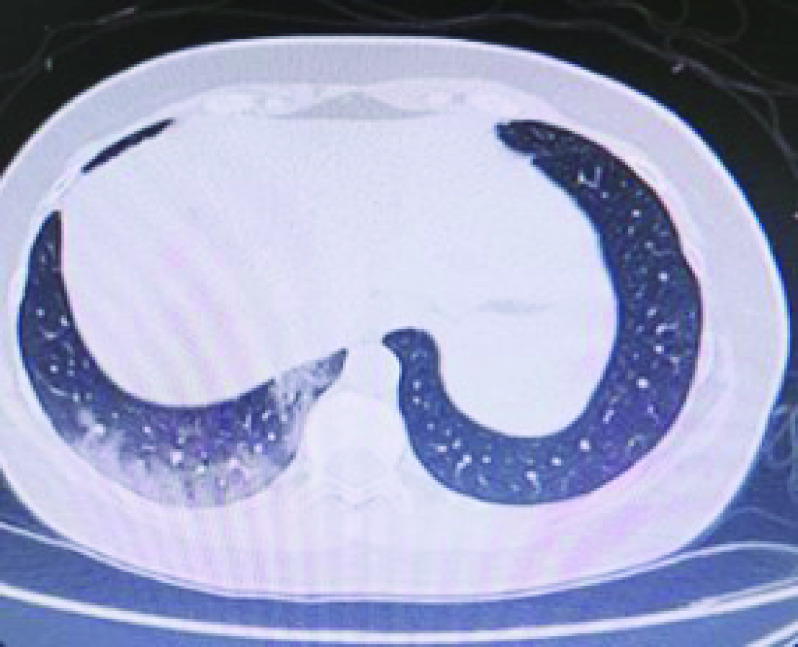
Thoracic computed tomography scan, 15 November 2020.
